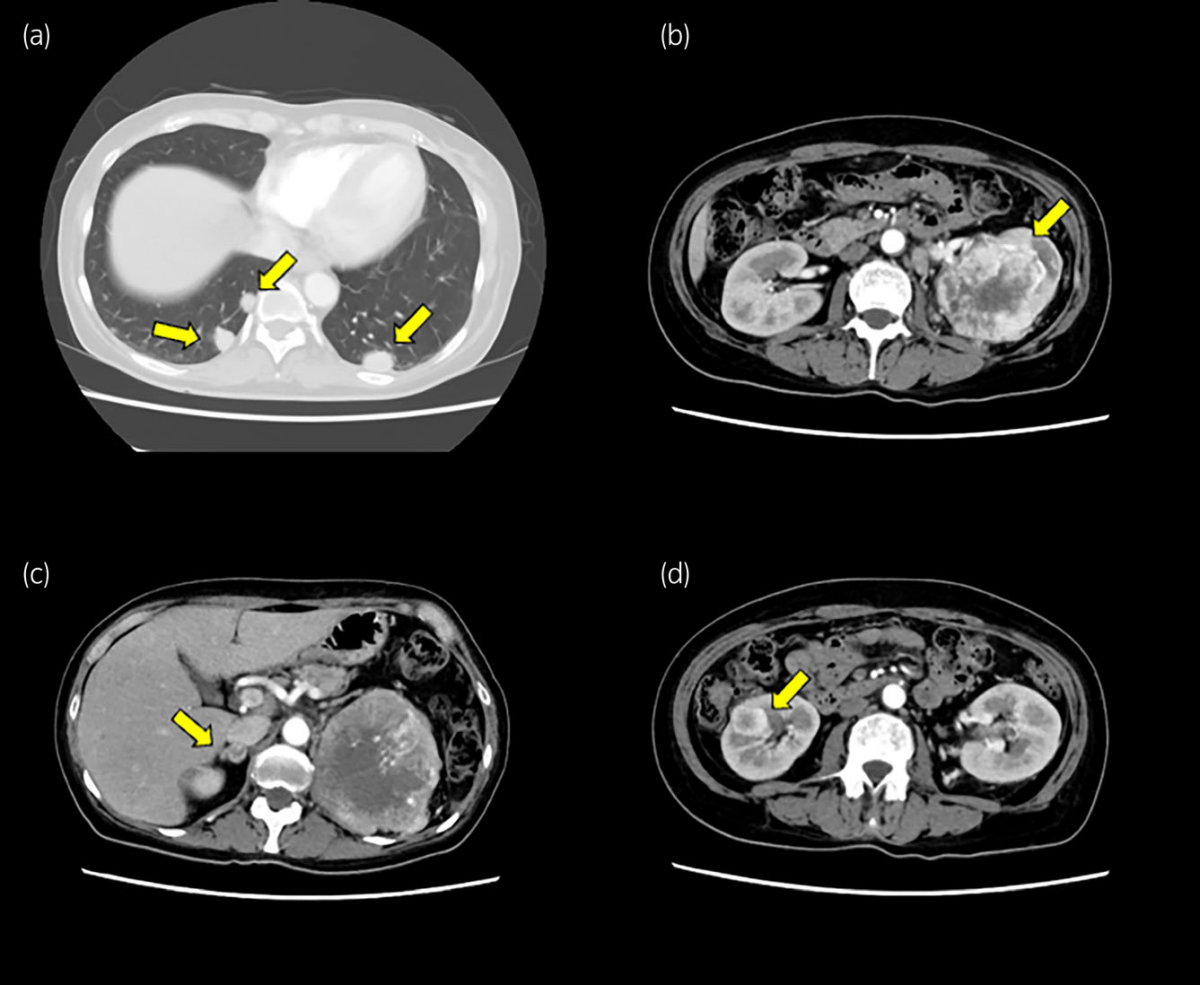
CT images. Contrast‐enhanced CT image showed multiple lung metastases (a), left renal tumor (b), right adrenal metastasis (c), and right renal metastasis (d) (yellow arrows).
